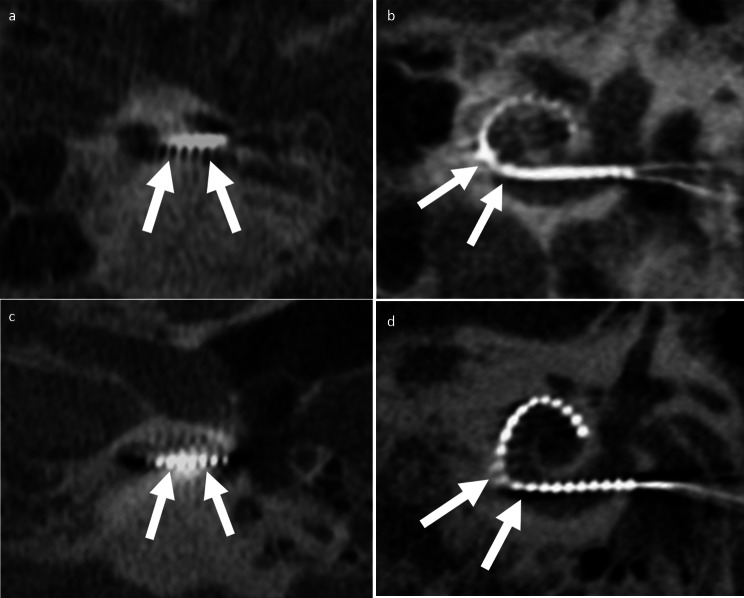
96-kV CBCT axial view of the basal turn of the cochlea (A) and a Stenvers projection (B) with inserted Slim-Straight CI522 electrode (Cochlear Limited) in patient with bilateral progressive sensorineural hearing loss (right ear shown). (C) and (D) representing 120-kV CBCT images of a patient with progressive sensorineural hearing loss with inserted Slim-Straight CI522 electrode (Cochlear Limited) on the left side. While 120-kV images had better delineation of electrode contacts (white arrows), all other evaluated image characteristics were rated similar between 96-kV and 120-kV
